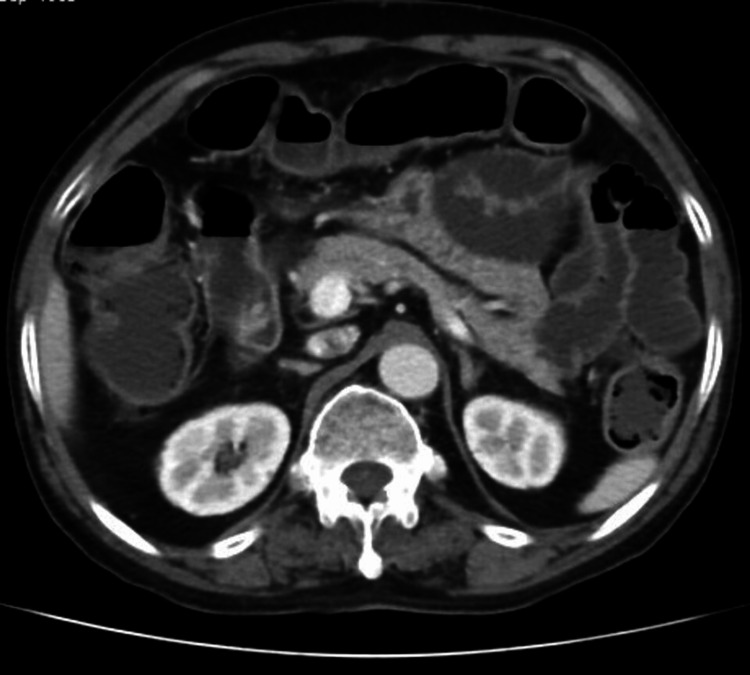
CECT of the abdomen showing normal pancreas morphology. CECT: contrast-enhanced computed tomography
